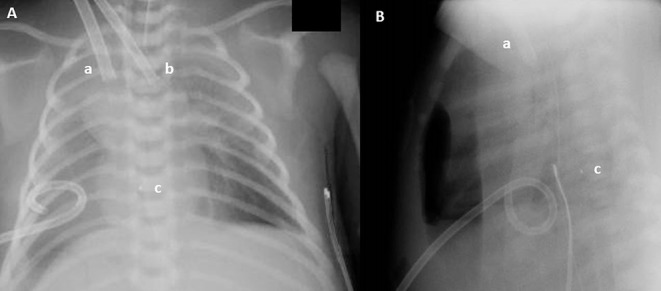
Anteroposterior (A) and lateral (B) chest X-ray: Drainage cannula (12 French) inserted in the jugular vein (a), inflow cannula (8 French) inserted in the carotid artery (b), and cannula tip (c). Even if in (A) the venous cannula seems to have a correct position, lateral chest X-ray reveals that the tip (c) of the venous cannula lies posteriorly to the heart.
